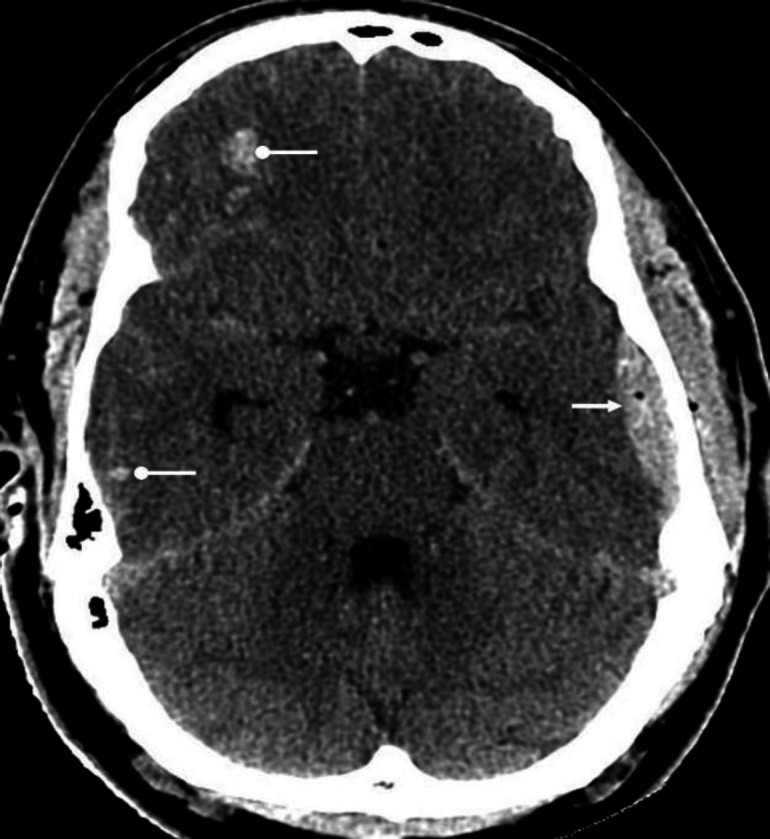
Non-contrast CT brain of a 24 year-old male following a road traffic incident. The bi-convex hyperdensity in the left temporal region (white arrow) is suggestive of an epidural haematoma. Note multiple foci of cerebral contusion (white circle-head arrows) that appear as focal hyperdense areas in the right frontal and temporal lobes
